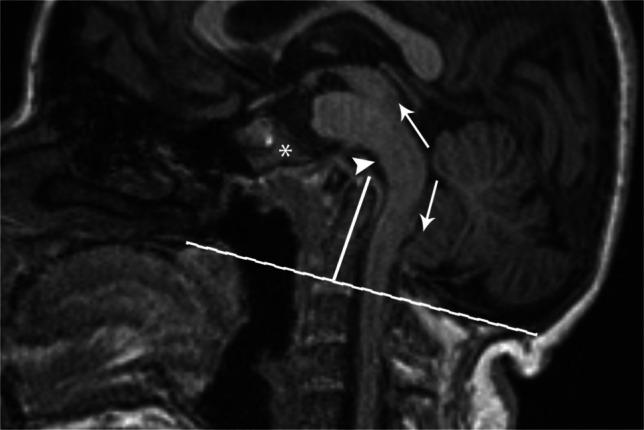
Sagittal T1 MRI in a 17-year-old female. Platybasia, clival hypoplasia (asterisk), and basilar invagination are present. The dens (solid white line) extends >4.5 mm above the McGregor line (dashed line). Imaging appearance does not always correlate with symptoms. Despite the severe imaging appearance, in this specific case, the patient was asymptomatic at the time of imaging. In symptomatic patients, symptoms are primarily bulbar in nature and theorized to be due to one or more of the following mechanisms: direct mass effect on the ventral brainstem (arrowhead), distraction of the dorsal brainstem (arrows), or altered biomechanics at the craniocervical junction.
